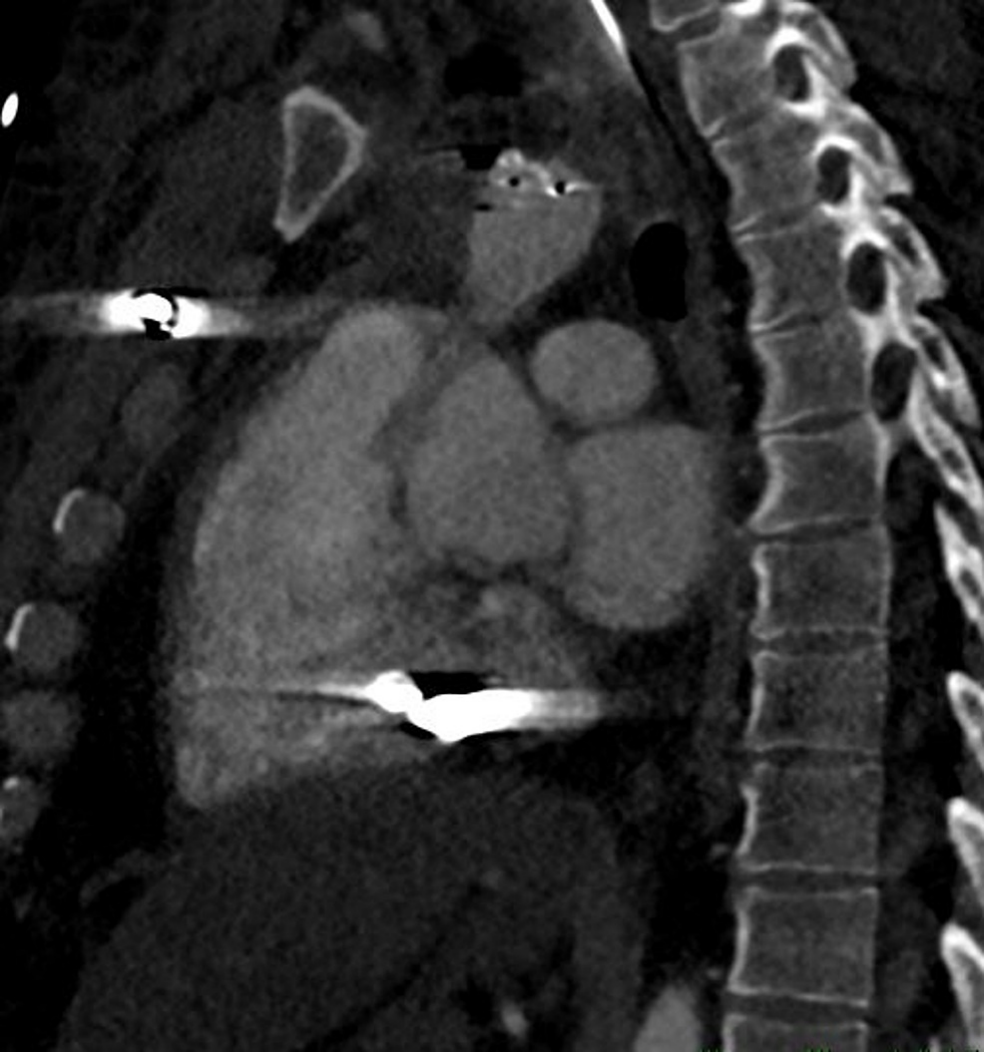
Lateral CT of the chest. Displays a hyperattenuated signal at the location of the bullet within the inferior aspect of the pericardium. Additionally, a second bullet is visualized by the hyperattenuated signal in the anterior thorax. CT: computed tomography
